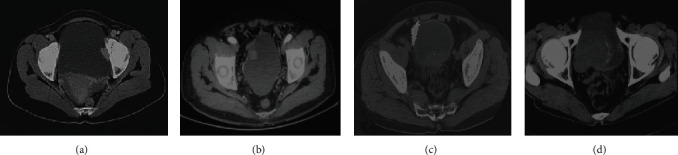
CT scan images of bladder cancer staging effects. (a)~(d) showed the images in stages T1, T2, T3, and T4, respectively.
